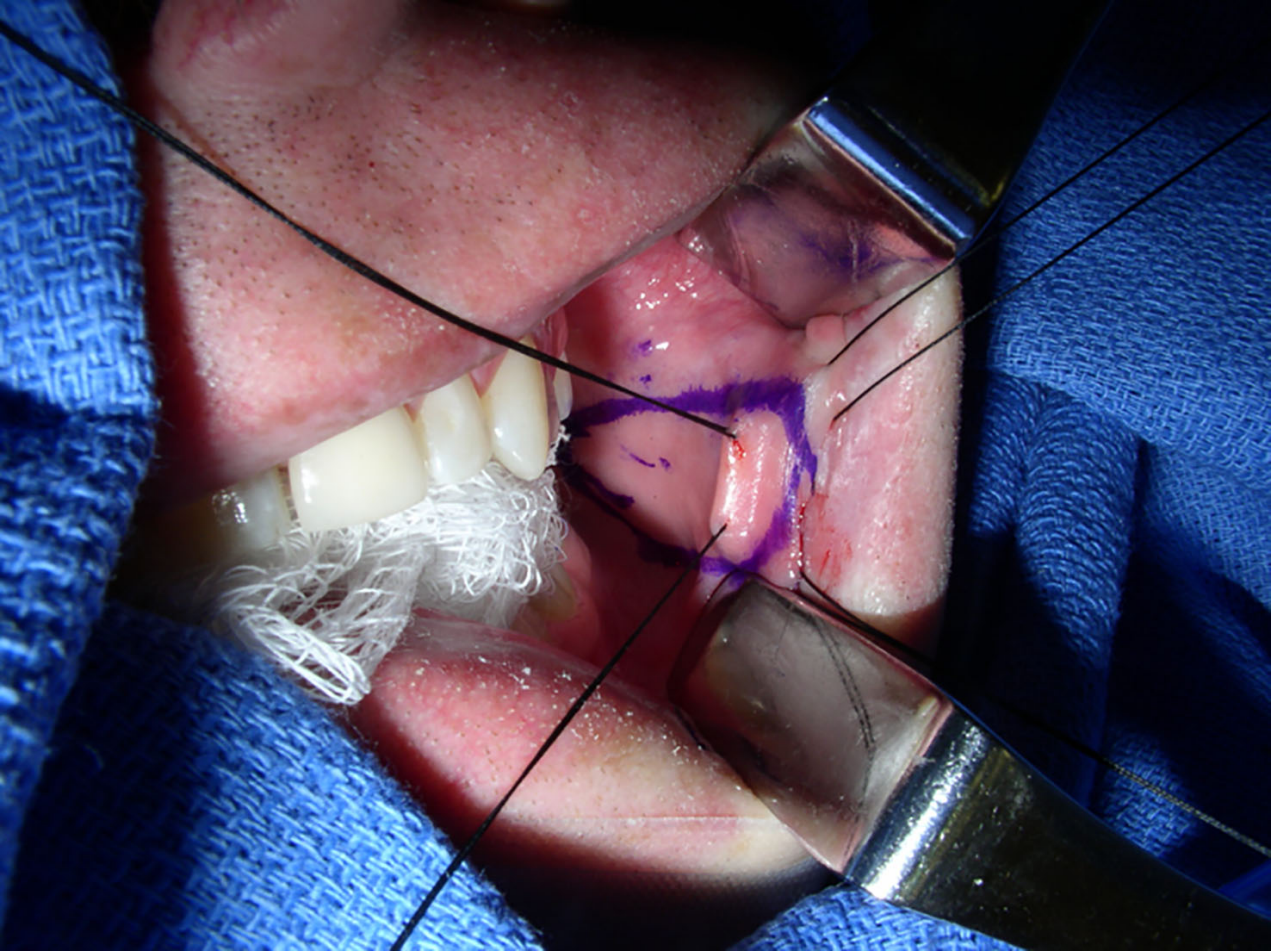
Typical setup for BMG harvest. The dry X-ray-detectable gauze sponge is packed to the contralateral oral space, and three retraction sutures are used with the middle incorporated into the distal apex of the graft. The Army–Navy retractors were used only for photographic exposure of the harvest site and are not typically needed during harvest. The image was obtained intraoperatively at Duke University Medical Center, with the operation completed under routine care. BMG, buccal mucosal graft.
